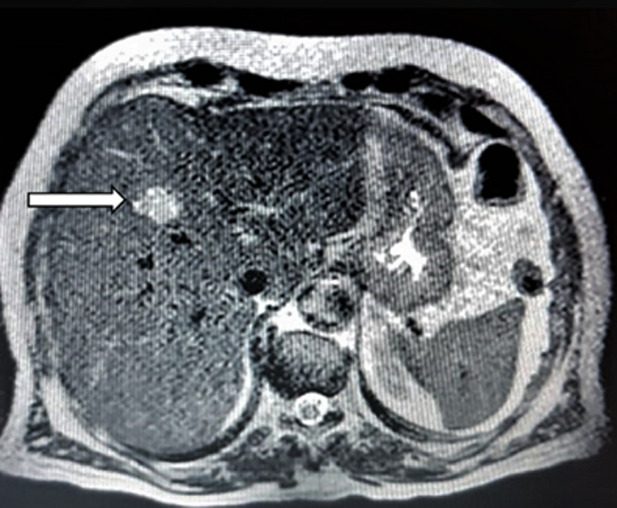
magnetic resonance imaging showing metastatic hepatic lesion 26mm in the IV segment (arrow)
